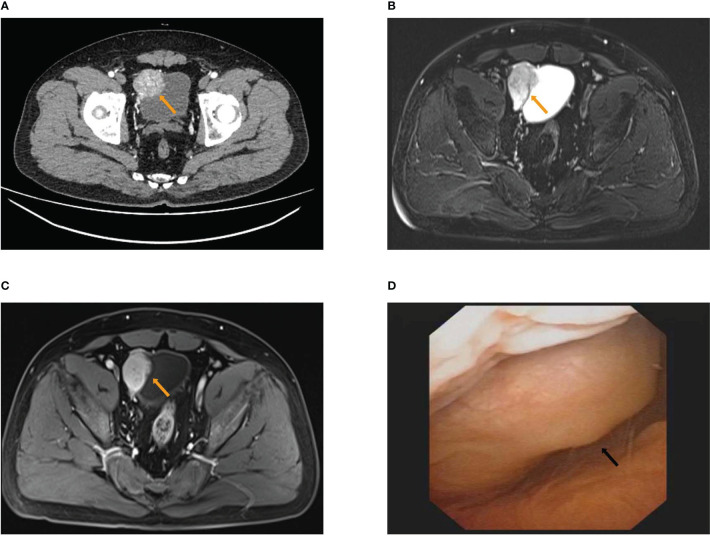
The results of preoperative examination. (A) The computed tomography urography (CTU) scan showed a roundish soft tissue lesion (4.8 cm × 3.7 cm) in the right anterior wall of bladder with heterogeneous enhancement of arterial phase. (B, C) MRI showed an approximately circular signal of high T2WI (4.9 cm × 3.8 cm) in the right anterior wall of bladder (B) with heterogeneous enhancement of arterial phase (C). (D) Cystoscopy showed inward right anterior wall.
